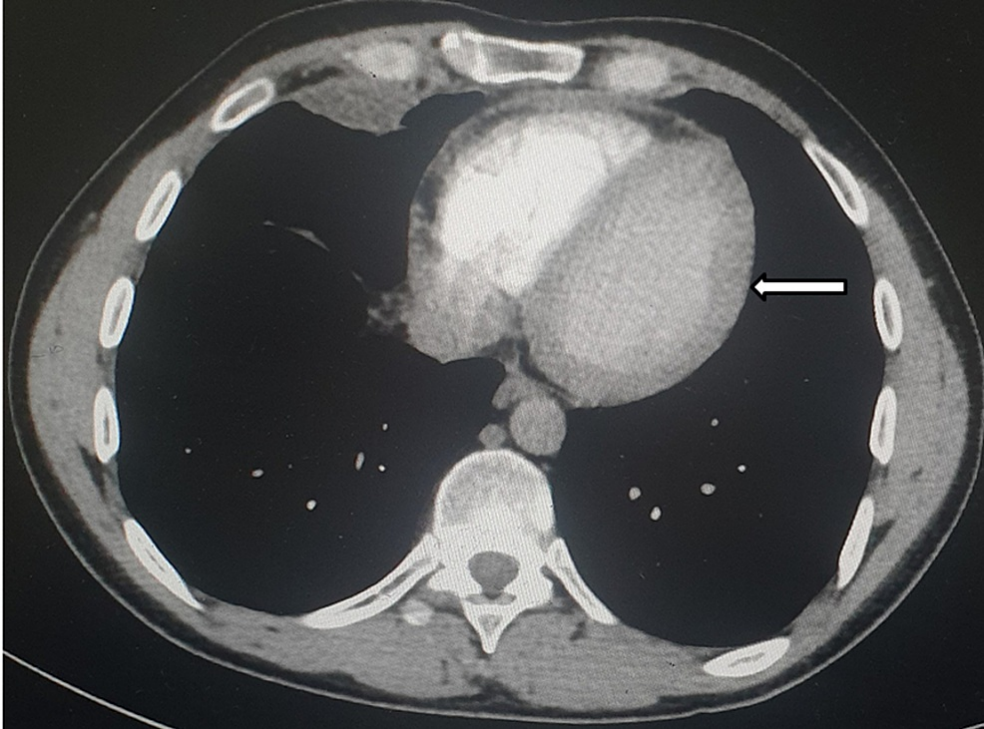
CT scan demonstrating mild pericardial effusion (white arrow)
